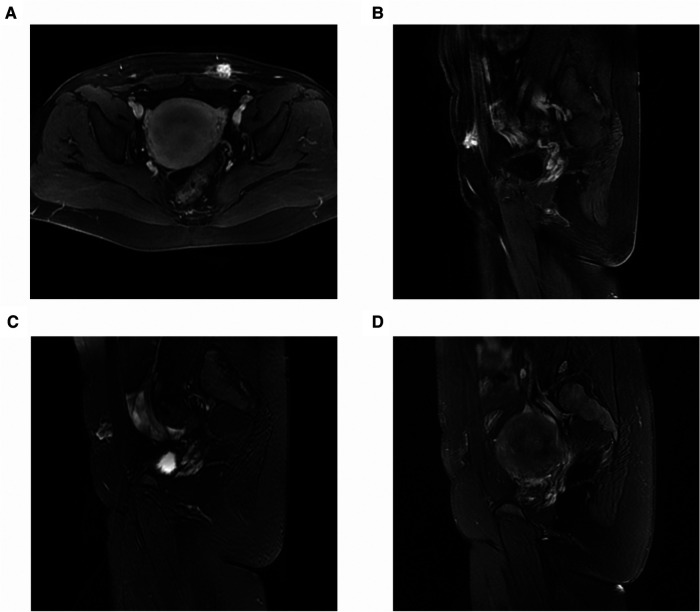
MRI of a 31-year-old patient with abdominal wall endometriosis treated with ultrasound-guided microwave ablation. Before microwave ablation, MRI showed remarkable enhancement on enhanced fat-suppressed T1-weighted axial images (A) and sagittal images (B), and inhomogeneous high-signal intensity on the T2-weighted sagittal images (C). After treatment, coagulation necrosis in the lesion area indicated that there was no residual enhancement at the treatment site and that the lesion had decreased in size (D). MRI, magnetic resonance imaging.
